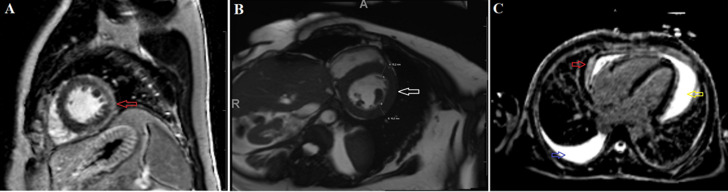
cardiac MRI examples of cardiac diseases that occurred in the audit: A) myocarditis: subepicardial delayed gadolinium enhancement (red arrow) of the anterolateral, posterior, and inferior left ventricular wall on a high-resolution short-axis phase-sensitive inversion recovery sequence is consistent with myocarditis according to the Lake Louis 2018 criteria; B) hypertensive heart disease, concentric left ventricular wall hypertrophy (white arrow) on a short-axis steady-state free precession sequence; C) tuberculous pericarditis confirmed on pericardial fluid aspirate, a large pericardial effusion (yellow arrow) with a thickened and enhancing pericardium (red arrow) on a four-chamber delayed gadolinium enhancement phase-sensitive inversion recovery sequence is consistent with acute tuberculous pericarditis; a right pleural effusion is also present (blue arrow)
